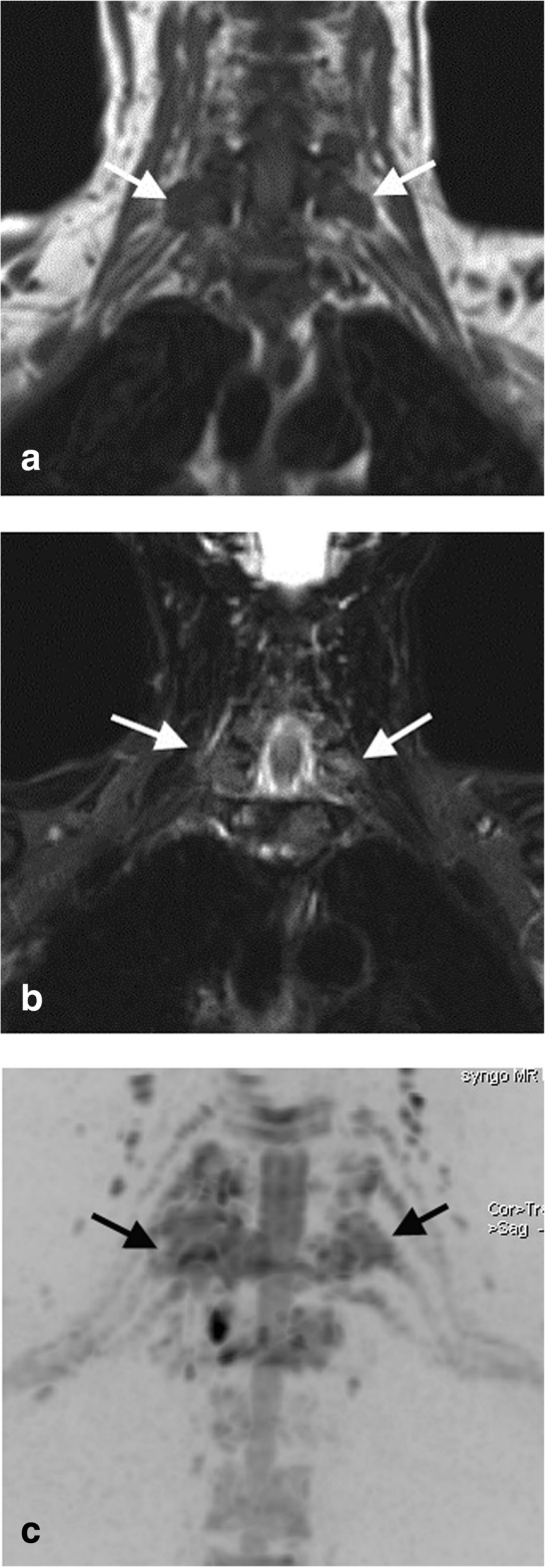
A48 year-old women with breast cancer with right arm symptoms of pain and numbness. (a) Coronal T1-weighted, (b) coronal STIR and (c) coronal inverted greyscale maximum intensity projection (MIP) of b = 700 s/mm^2^ images. On the MIP diffusion images, note abnormal impeded diffusion involving the right ganglia and nerve roots of C6 to C8, and also C7 on the left (black arrows). The disease was missed by one reader on the conventional imaging (white arrows).
